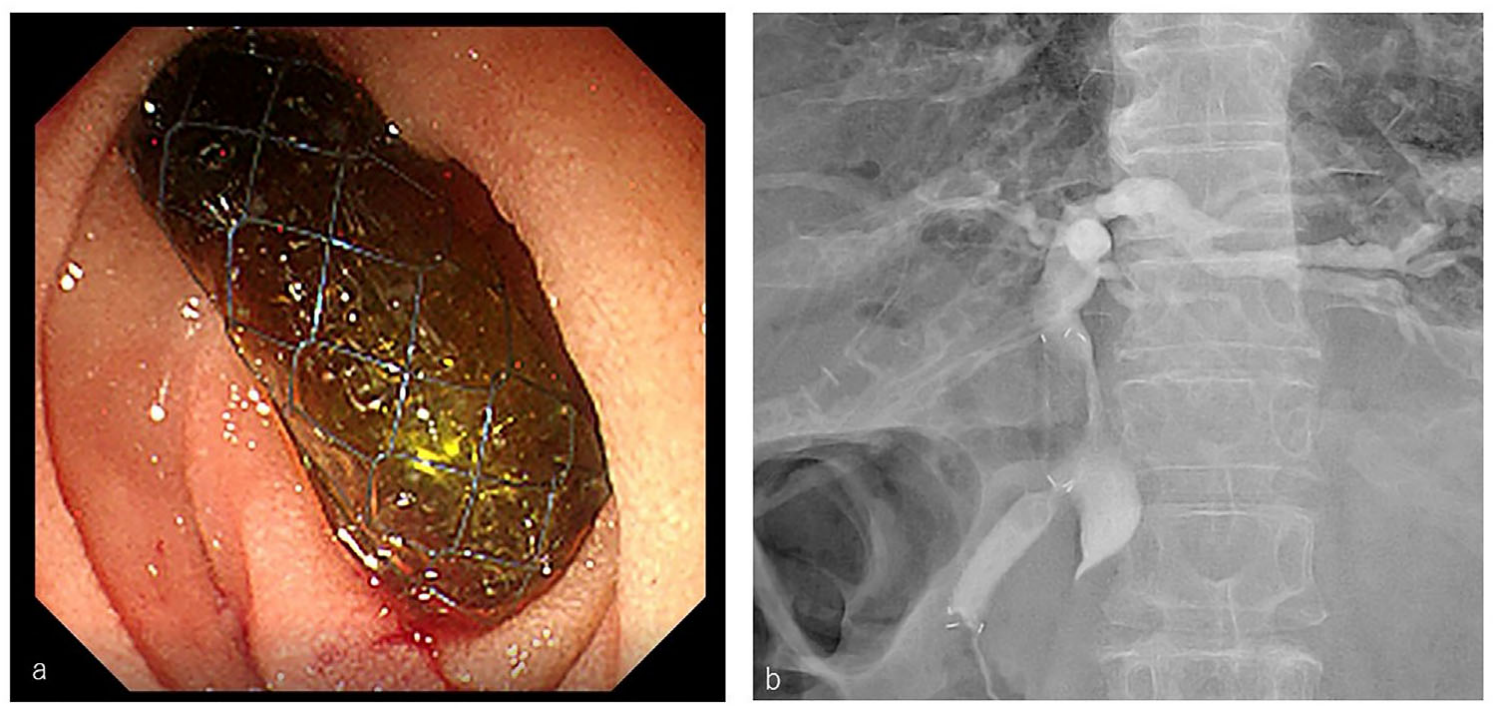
Imaging findings following endoscopic ultrasound‐guided choledochoduodenostomy without fistula dilation. (a) Endoscopic image. (b) Fluoroscopic image
